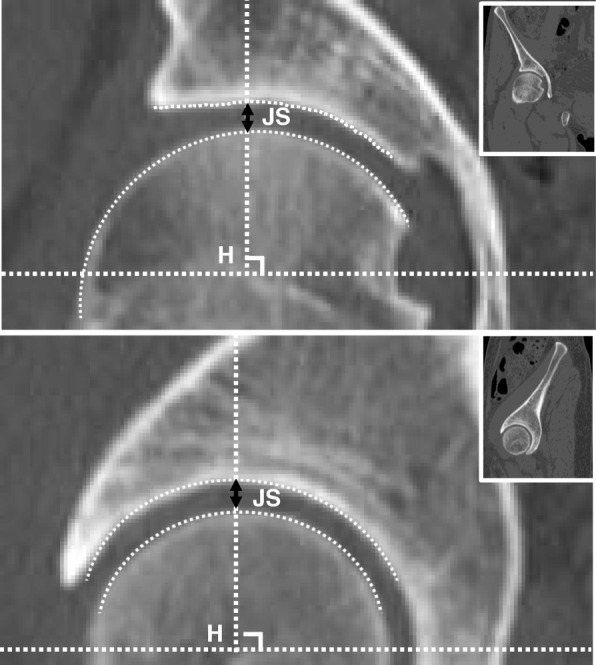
Method of joint space measurement by multiplanar reconstruction computed tomography (CT). The calculations of the joint space (JS) were performed between the surface of the cortical bone of the acetabulum and that of the femoral head on the perpendicular line from the centre of the femoral head (H). The appropriate point of sagittal and coronal views was determined at the slice where the size of the femoral head was most prominent. The upper row indicates the coronal view of mCT, and the lower one indicates the sagittal view. The wickets indicate a wider view of mCTs
